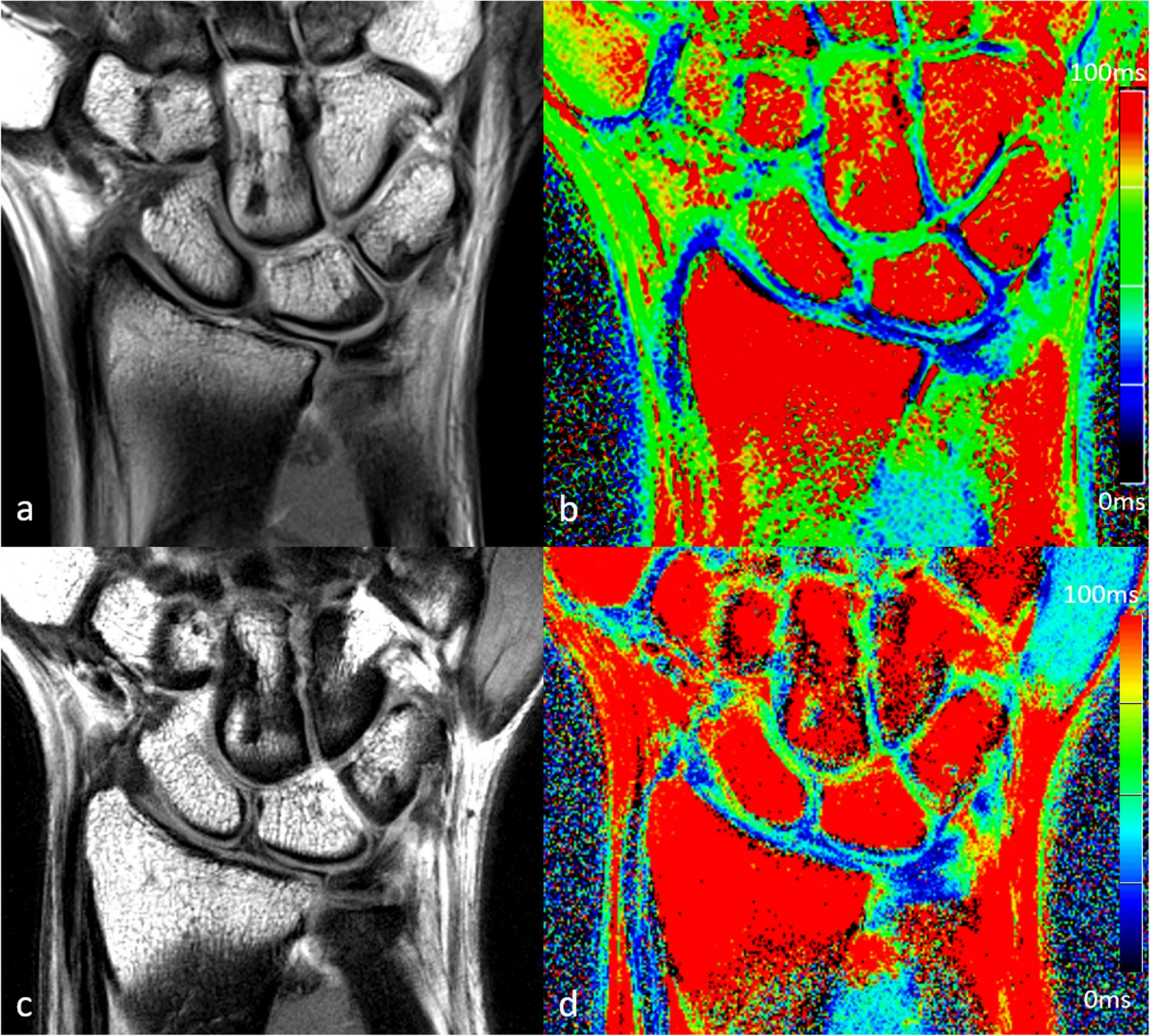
Three-Tesla (a, b) and 7-T (c, d) images of the first echo of the T2-weighted multi-echo spin-echo sequence (a, c) with corresponding color-coded maps of T2 values (b, d) of a 42-year-old patient with chronic wrist pain
